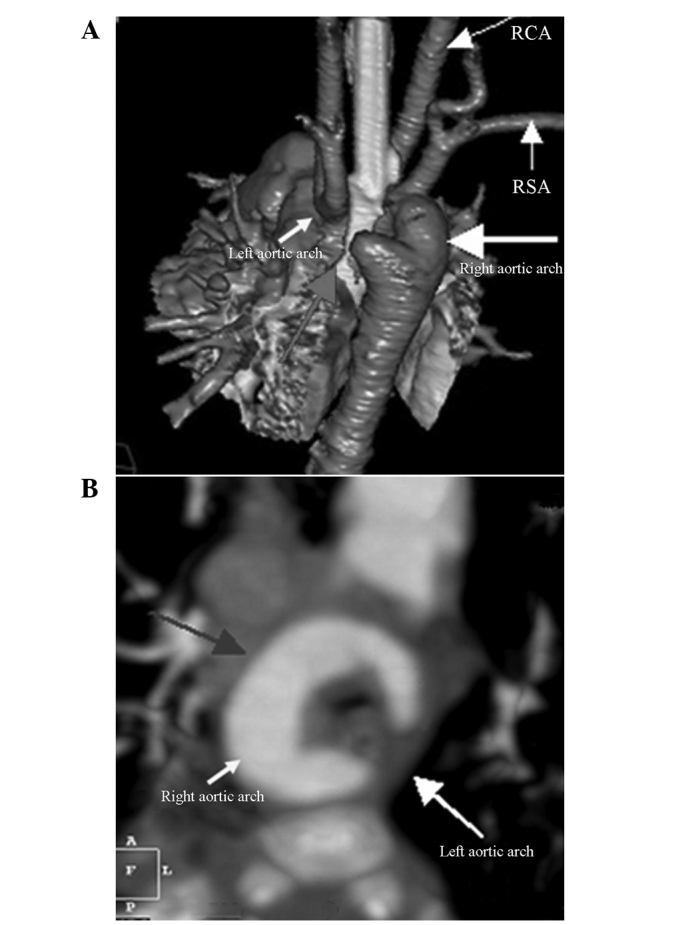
A three-month-old female infant with DAA. (A) The CT volume-rendered image shows that the aortic arch split into two arches surrounding the trachea (right thick arch and left arch atresia). (B) An axial CT section image. The aortic arch split into two arches surrounding the trachea and esophagus (left arch atresia and double arches show ‘C’ shape). DAA, double aortic arch; CT, computed tomography.
